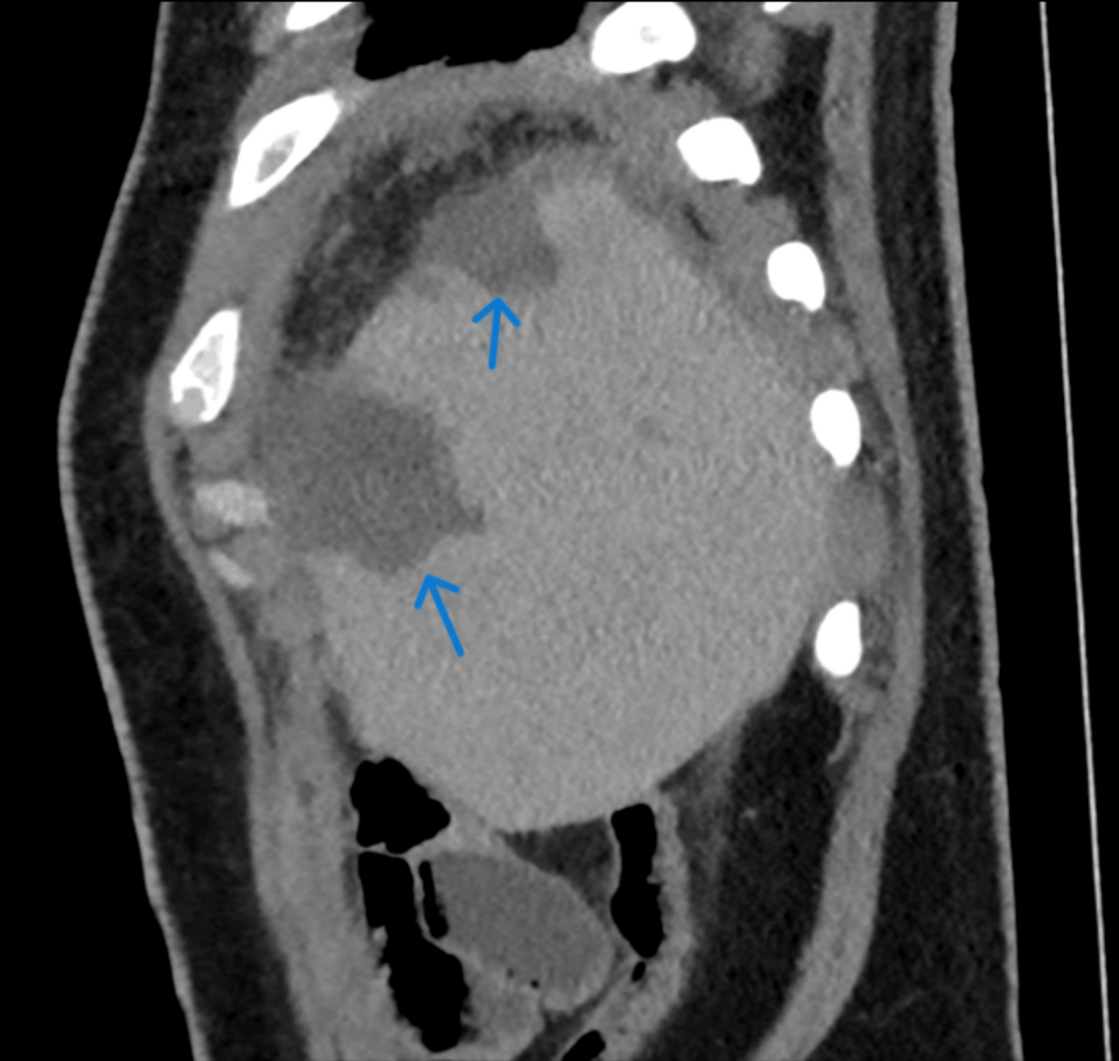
Sagittal reformatted CECT section shows enlarged spleen with peripherally based pyramidal-shaped infarcts in the splenic parenchyma (blue arrows) CECT: Contrast-enhanced computed tomography
